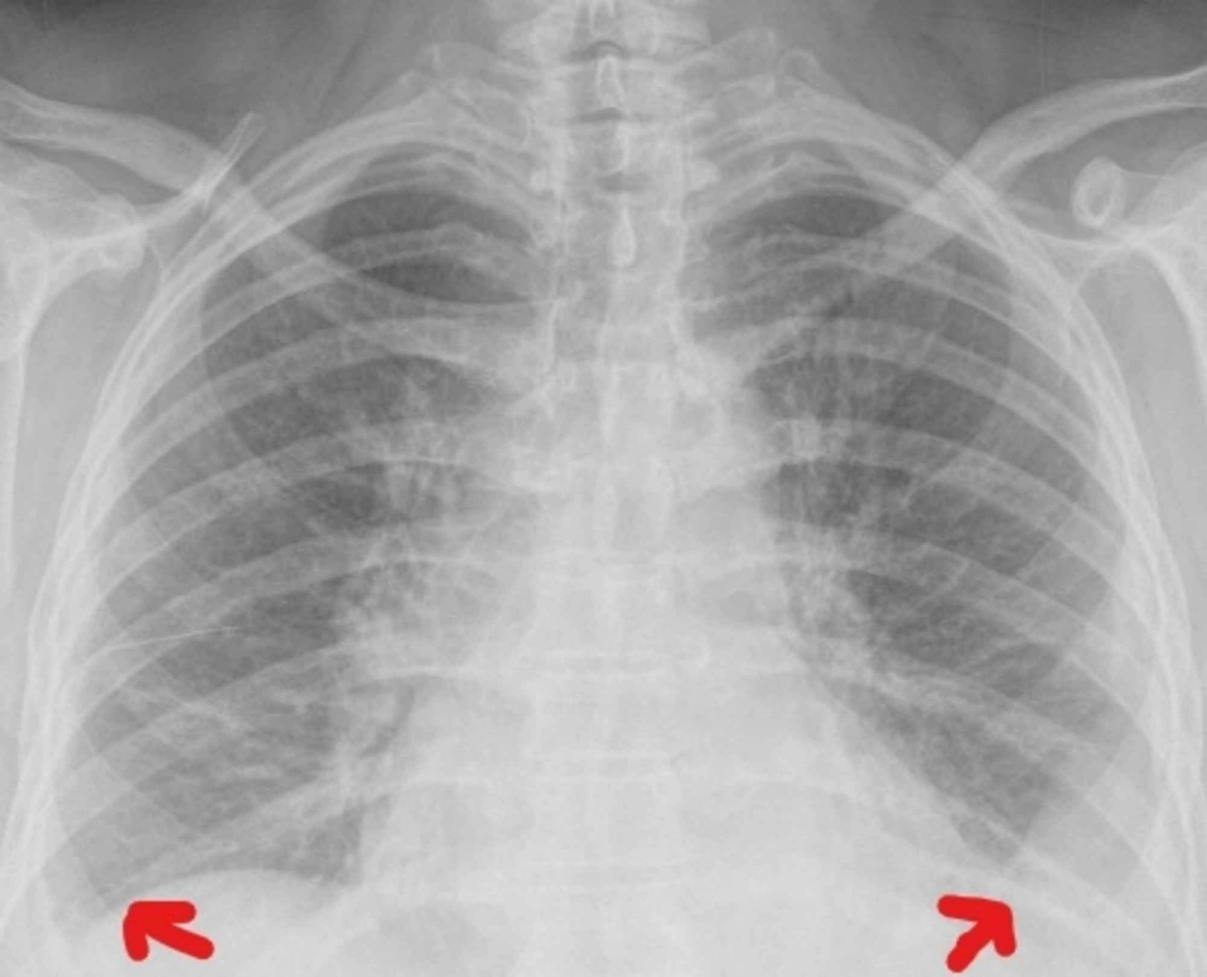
Thoracic radiography from day 11
